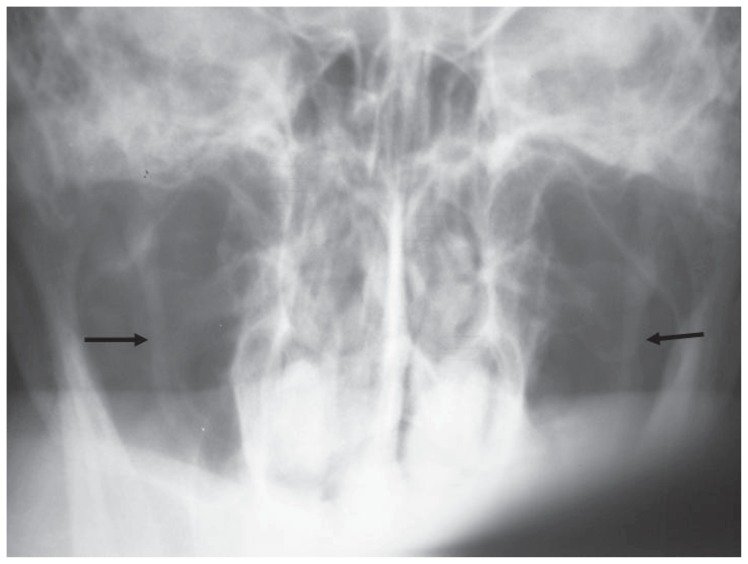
Towne radiograph shows bilateral elongated styloid processes (right: 44 mm, left: 44 mm).
